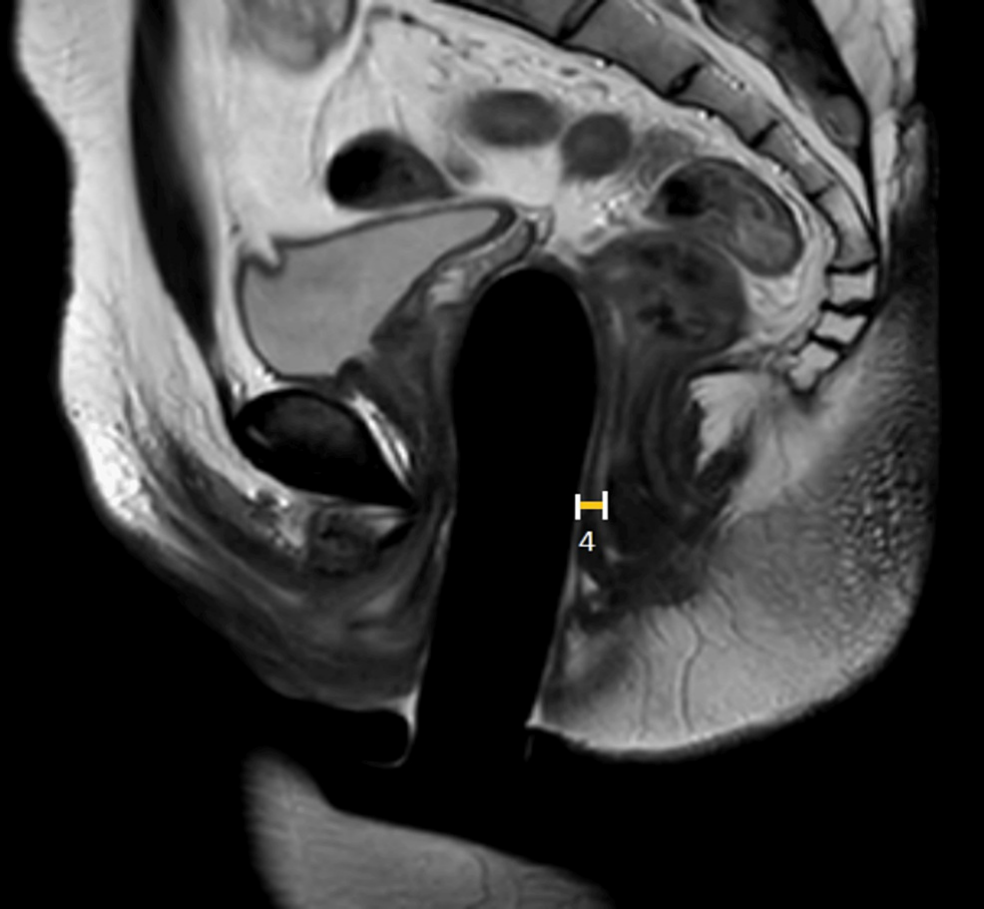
T2-weighted TSE sagittal MRI image of the post-operative patient Original MRI image of a patient who underwent the study. 4 - Rectovaginal thickness
